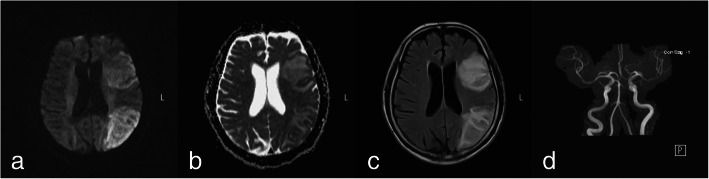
Brain magnetic resonance imaging on the fifth day after admission. a: Diffusion-weighted imaging (DWI) showed hyperintensity over the left frontal and temporoparietal regions. b: The apparent diffusion coefficient (ADC) image showed decreased ADC values in the same area. c: The T2-weighted fluid-attenuated inversion recovery (T2-FLAIR) image showed hyperintense regions in the same area. d: Magnetic resonance angiography (MRA) showed no obvious stenosis on the main trunk of the intracranial vessels. These findings combined with the clinical picture were consistent with acute ischaemic infarction
